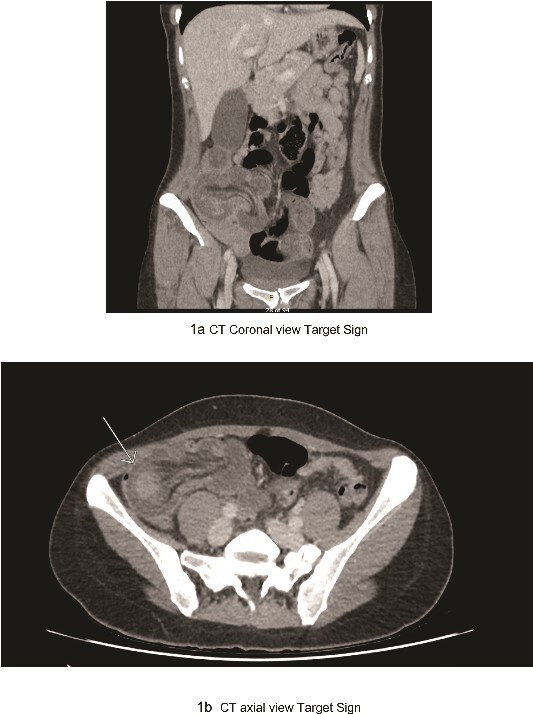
(a) CT coronal view target sign; (b) CT axial view target sign.
